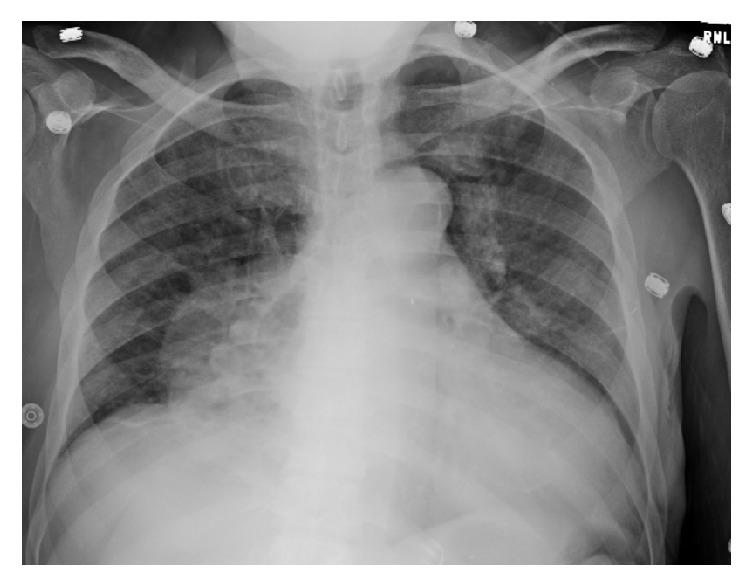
Chest X-ray showing a pneumopericardium, most probably due to the direct extension of the fungal infection (day 15).
